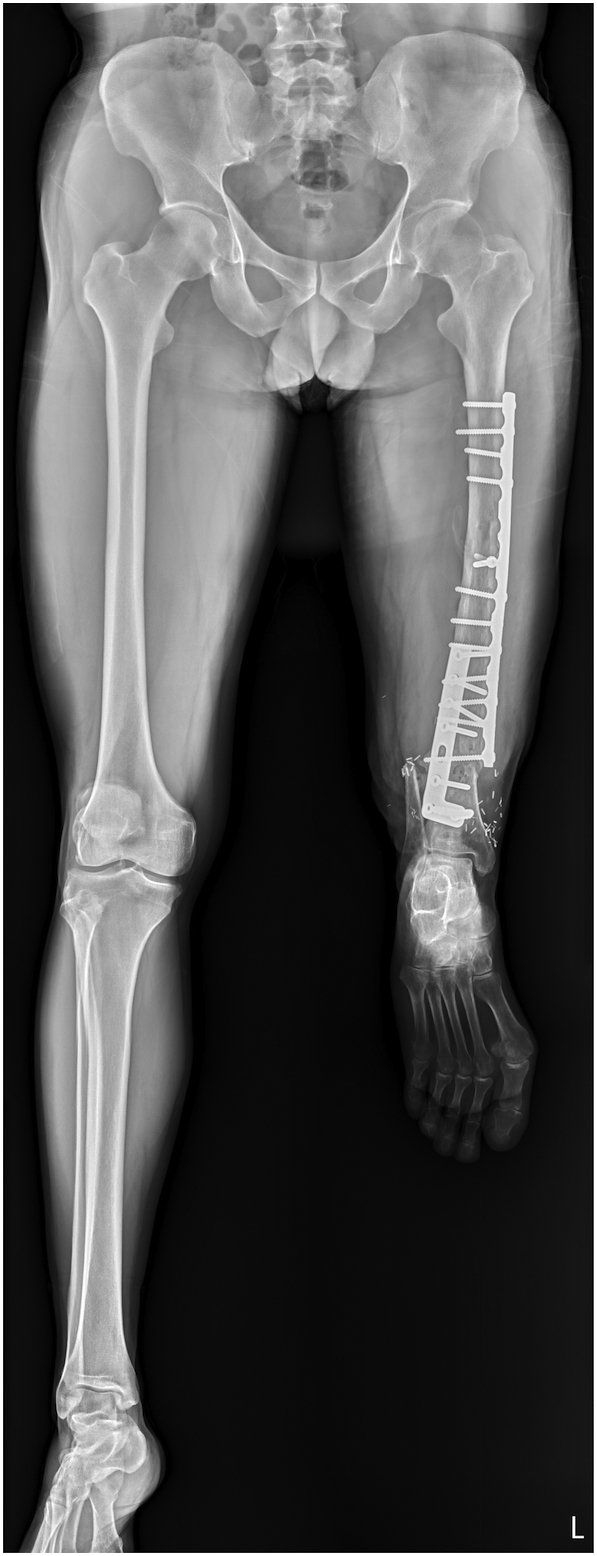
Whole leg axis radiographs. The bone union site has completely healed, and the axis of the leg is straight.
